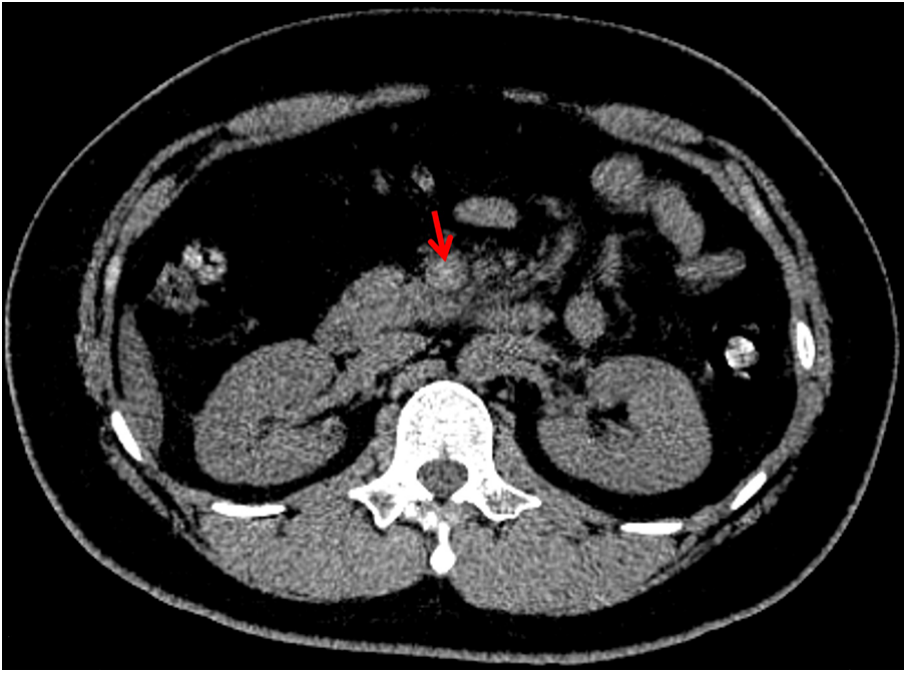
Unenhanced CT image showing high density lesion in superior mesenteric vein.
